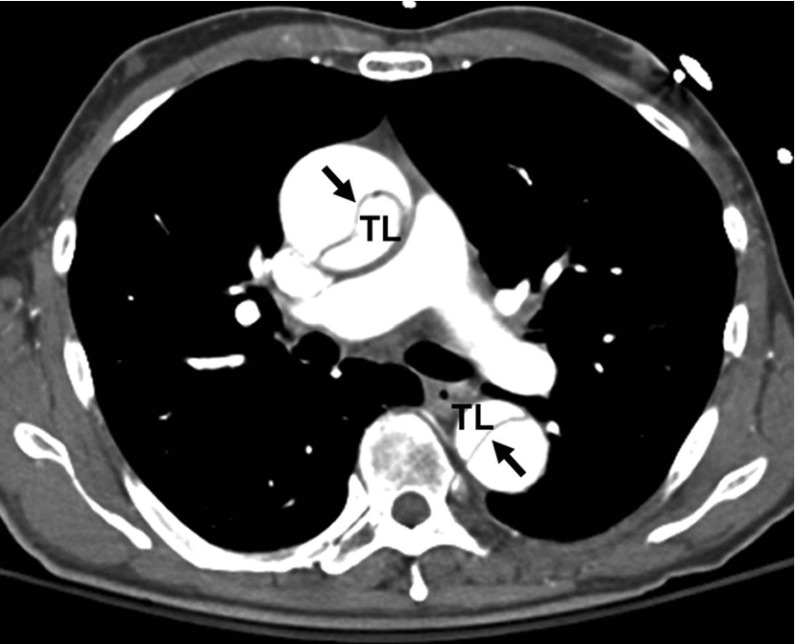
This CT shows an acute aortic dissection in the ascending and descending aorta (Fig. 2; Braverman 2010), with buckling of the inner wall indicated by the *arrows*. TL is the true lumen
